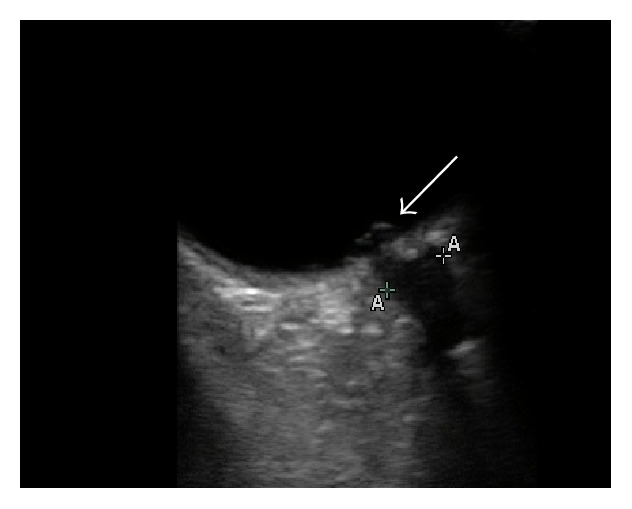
Bedside ocular ultrasound of the right eye demonstrating bulging of the optic nerve cup, indicative of papilledema.
